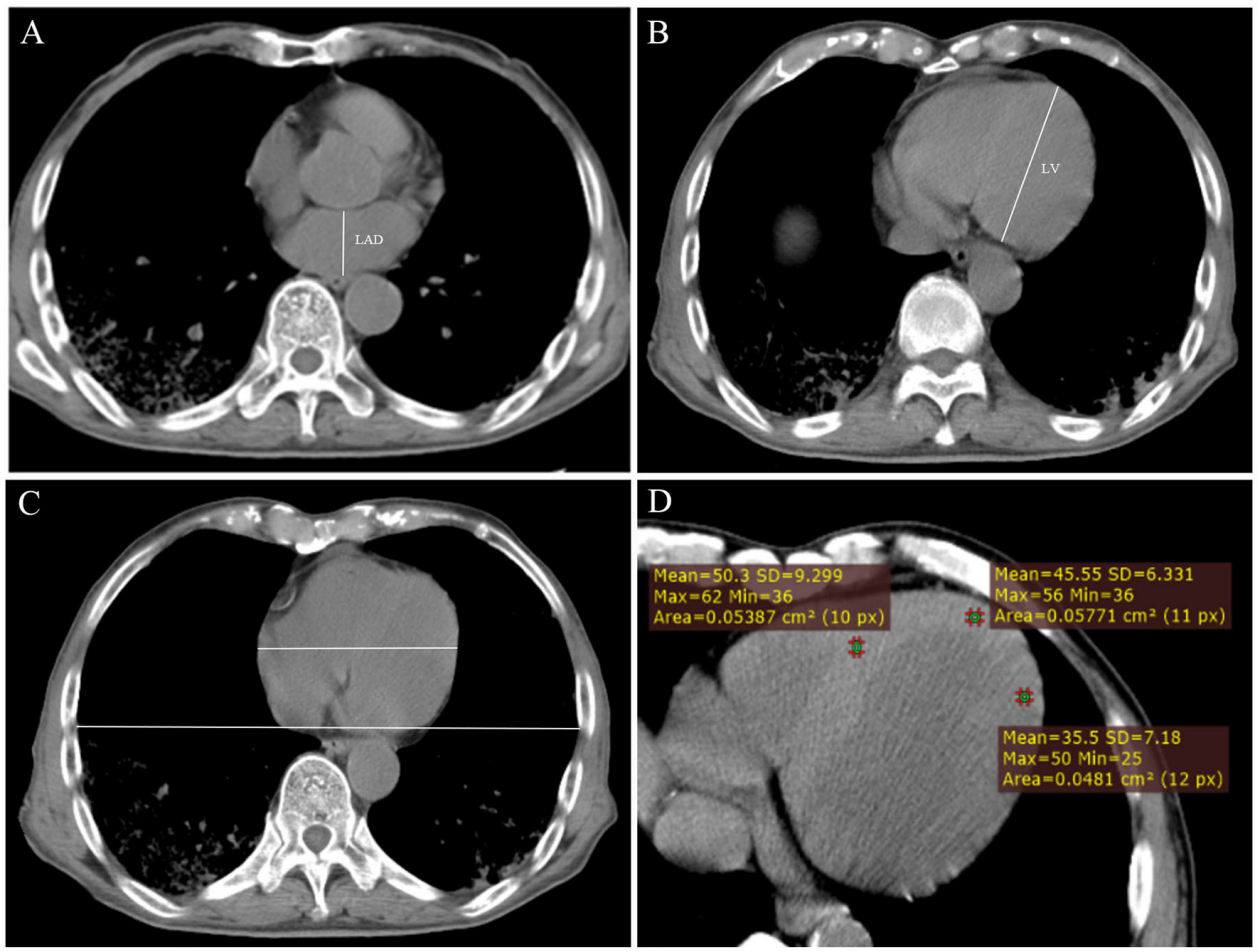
(A) Measurement of the anteroposterior diameter of the left atrium (LAD). (B) Measurement of the length of the left ventricle (LV). (C) Measurement of cardiothoracic ratio. (D) Measurement of CT value of myocardium at axial image.
